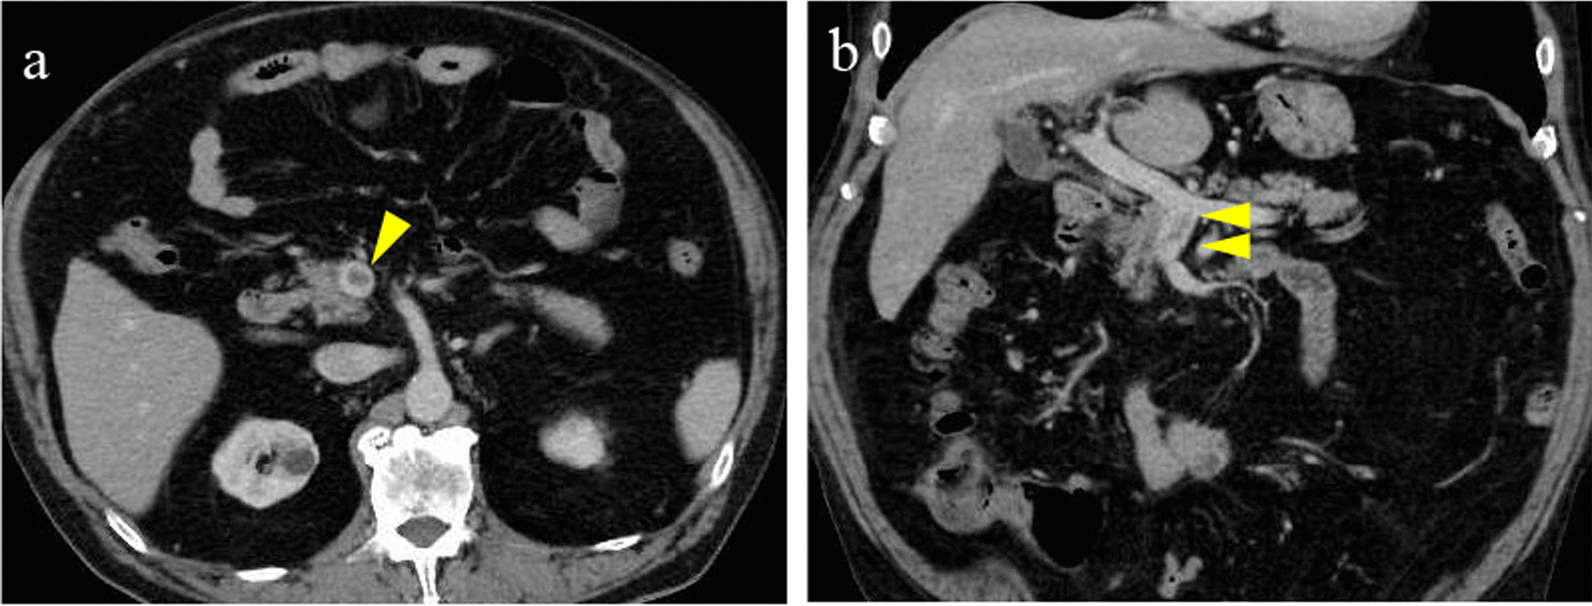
Abdominal computed tomography at 12 days after starting treatment. The SMV thrombus has shrunk, the ascites has disappeared (yellow arrows), and the small bowel edema and mesenteric adipose tissue density are improved after treatment. Axial image (a). Coronal image (b)
